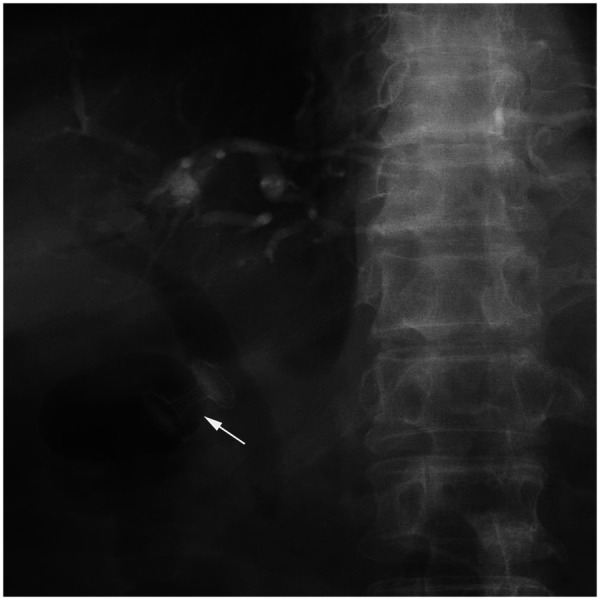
Abdominal X-ray showing a successfully implanted lumen-apposing metal stent (LAMS) (white arrow) between the duodenal bulb and common bile duct. The LAMS has a typical “hourglass” shape with bilateral flanges.
